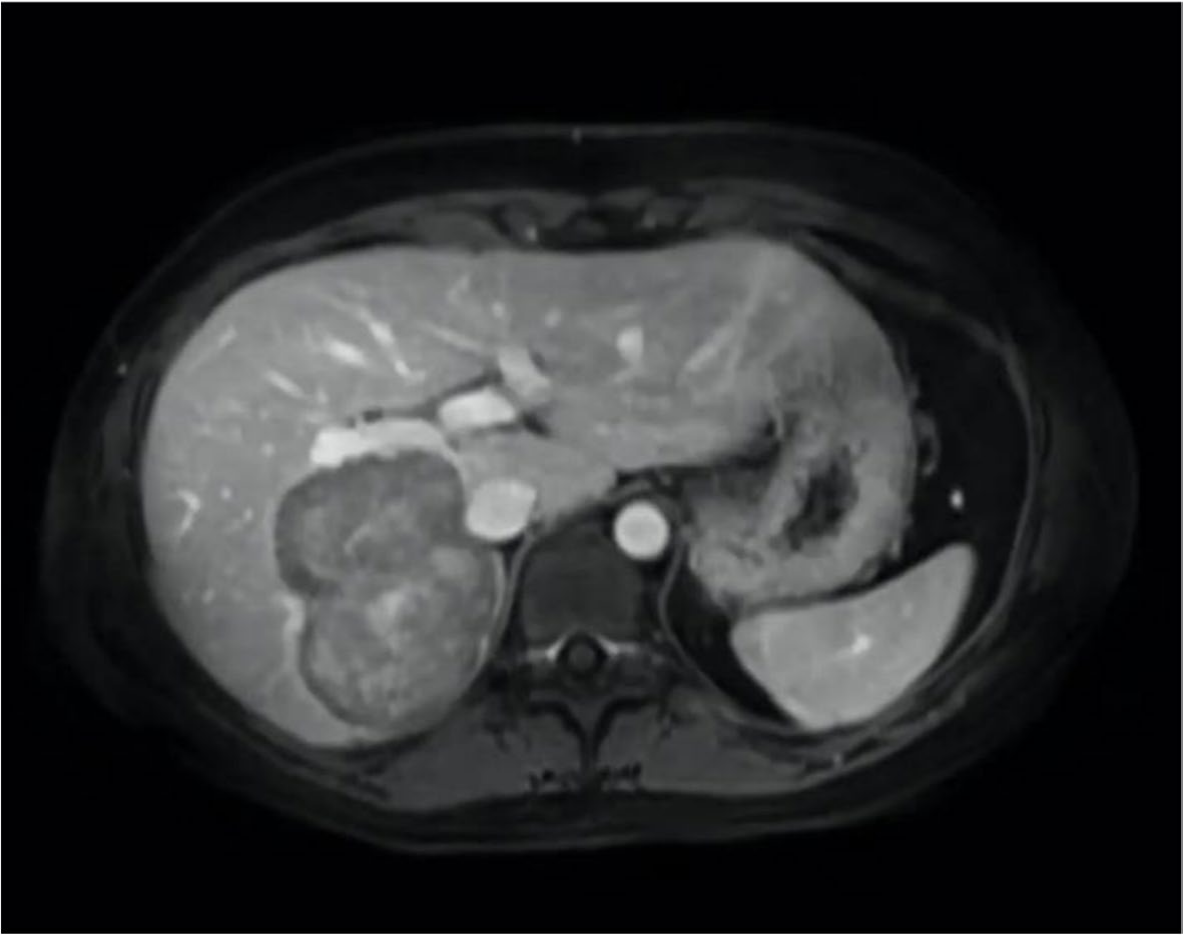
Magnetic resonance imaging of the patient with large adenoma
